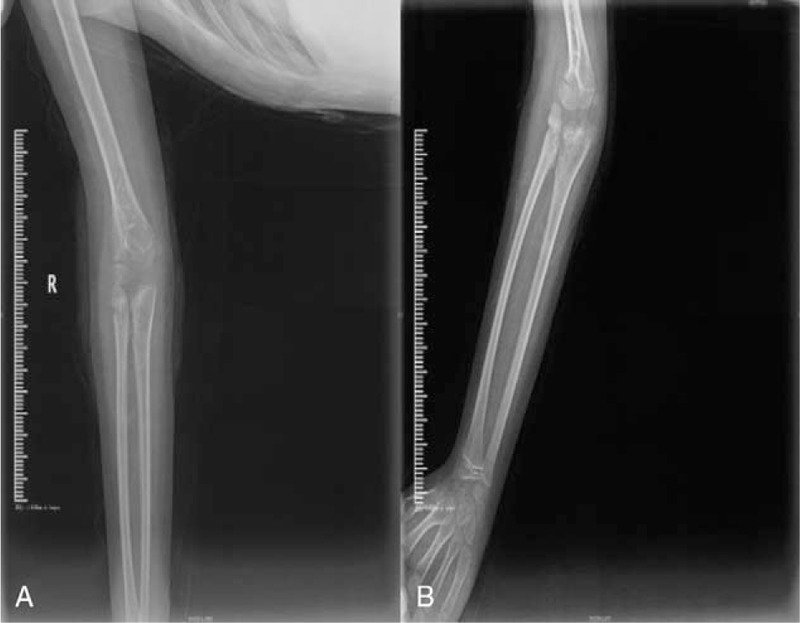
(A) X-ray of the anteroposterior elbow at the 4-wk postoperative follow-up. (B) X-ray of the lateral elbow at the 4-wk postoperative follow-up.
